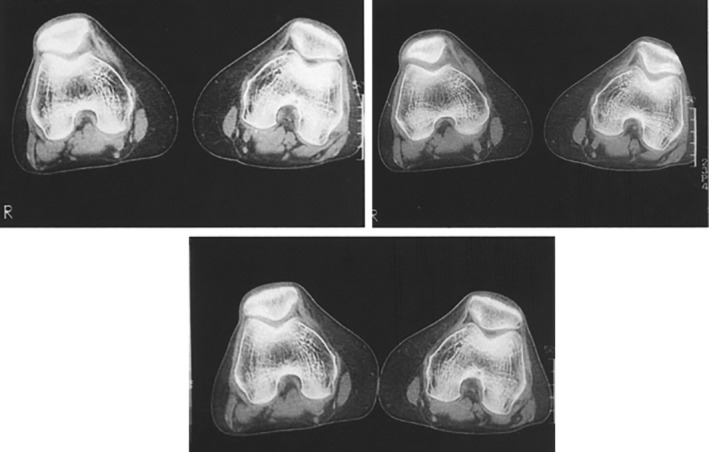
CT images obtained at different degrees of knee flexion and with/without quadriceps contraction^19^.
